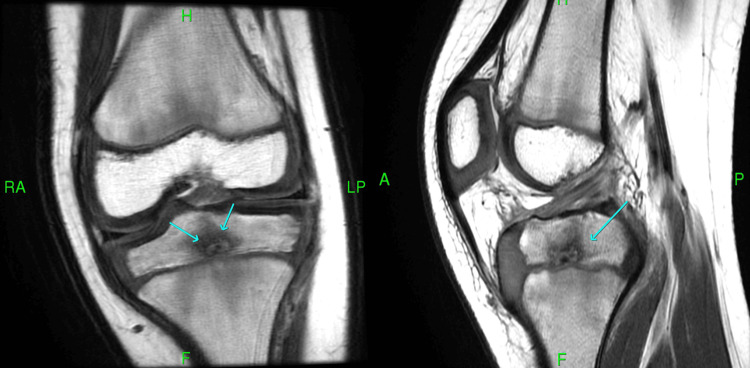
Coronal and sagittal T1-weighted MRI images showing persistence of the lesion (arrows) MRI: magnetic resonance imaging
